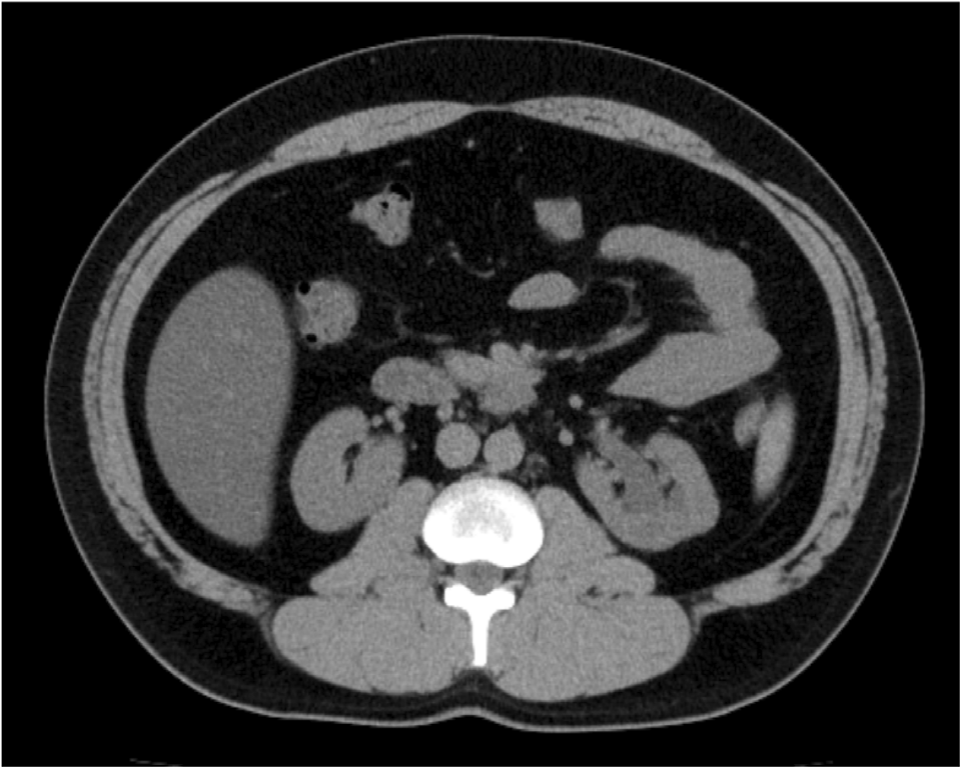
Postoperative computed tomography. Computed tomographic acquired approximately 2 years after surgery show improvement in hydronephrosis.
